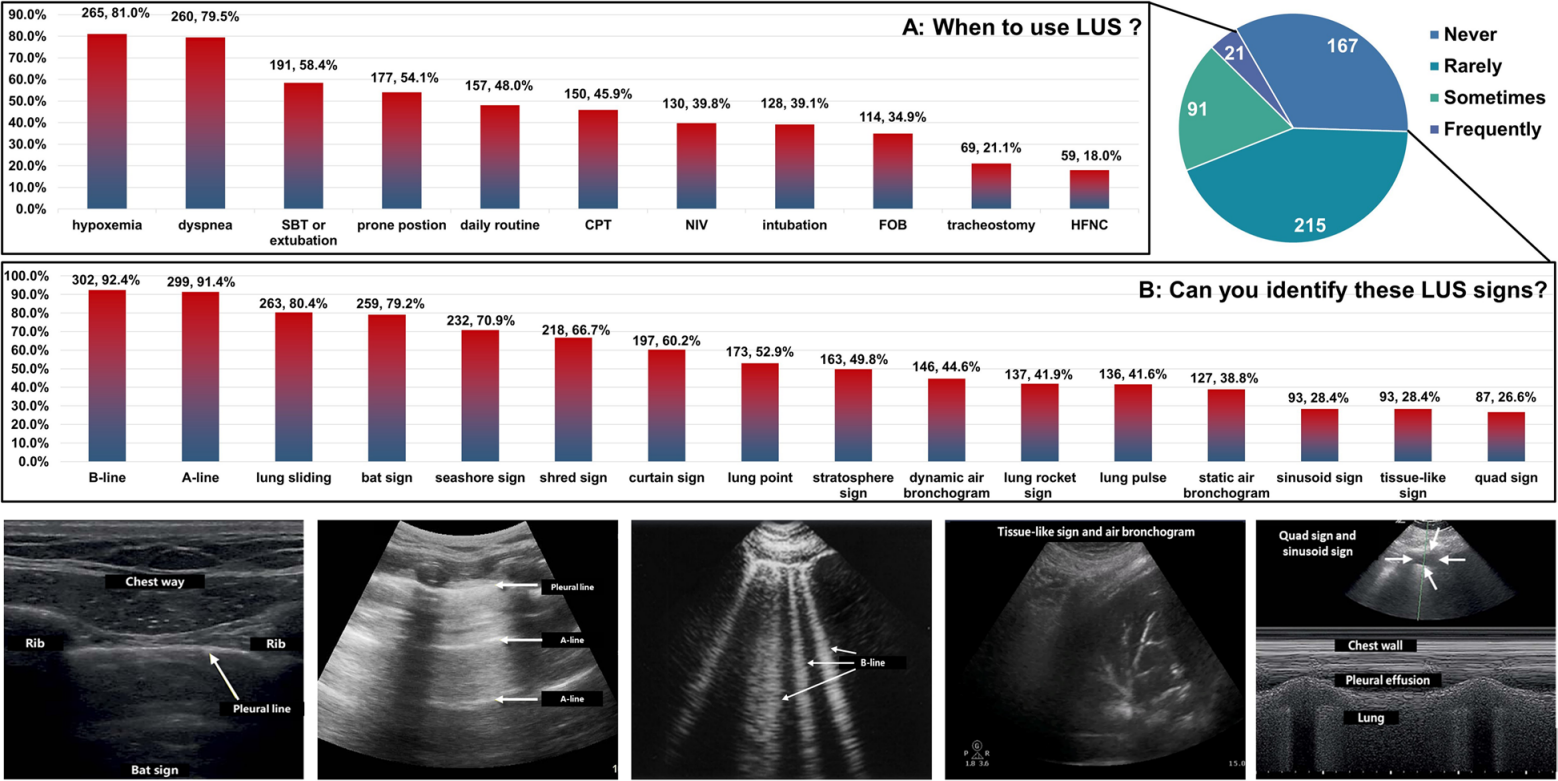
A When to use LUS? B Can you identify these LUS signs? LUS, Lung ultrasound; SBT: spontaneous breathing trial; CPT: chest physiotherapy; NIV: non-invasive ventilation; FOB fiber bronchoscopy; HFNC: high-flow oxygen therapy
